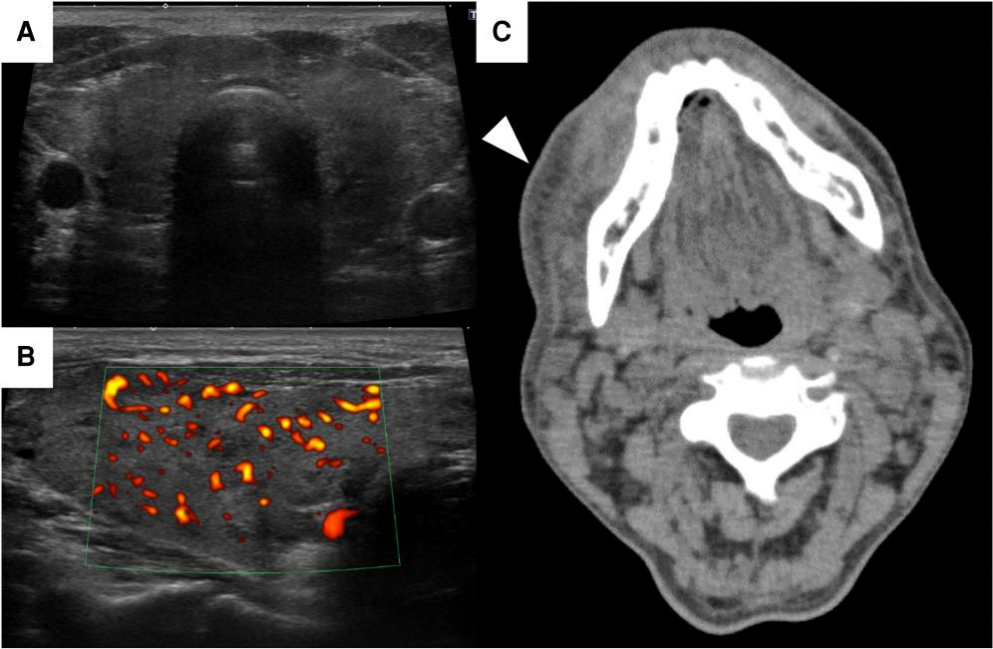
Transverse view (A) and power Doppler examination (B) of the thyroid ultrasonography. The thyroid appears enlarged and heterogeneous with increased vascularity on power Doppler imaging. Computed tomography of the head and neck (C). Soft tissue opacity is observed in the right buccal region (*arrowhead*).
